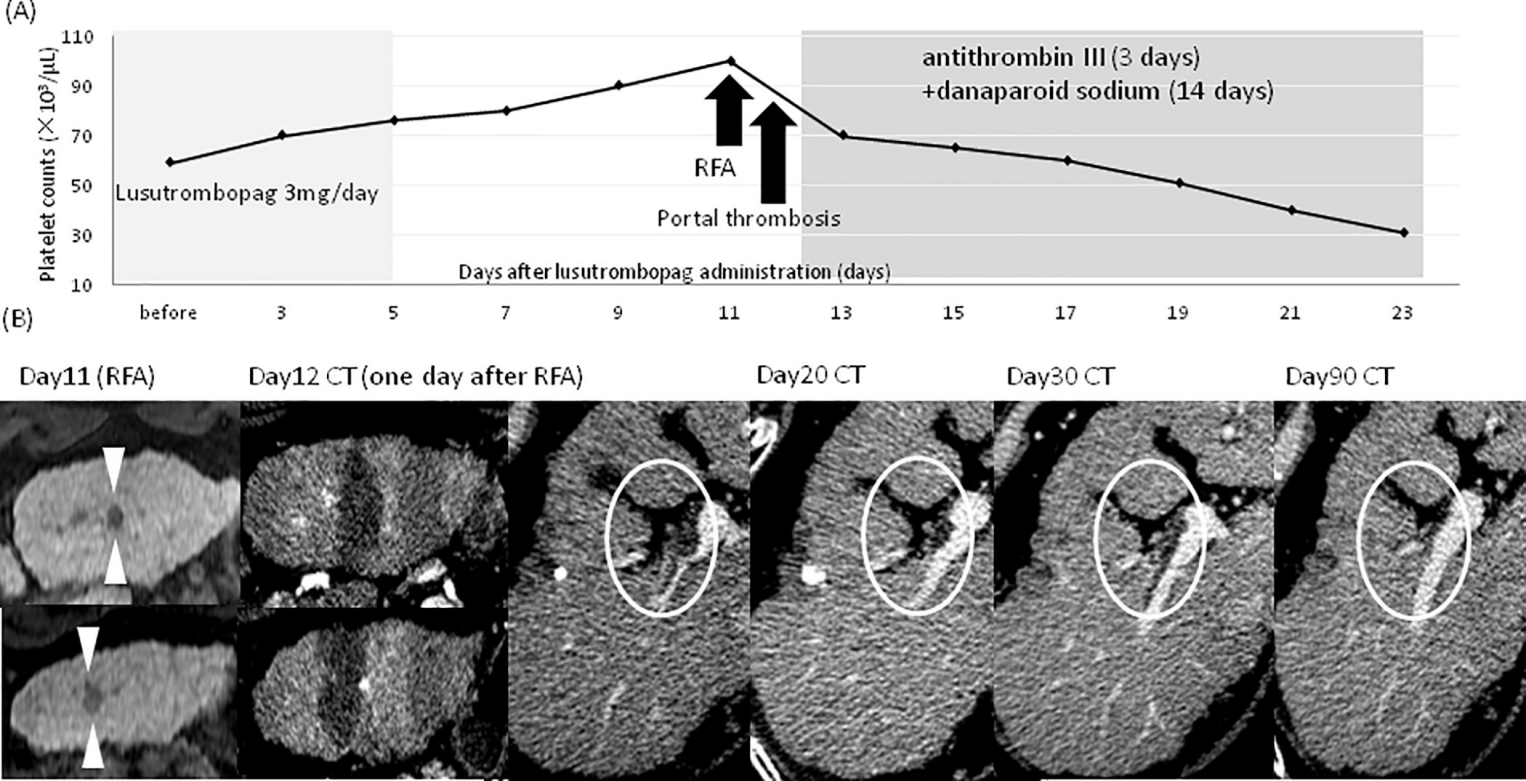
Detailed clinical course of a patient with portal thrombosis after lusutrombopag treatment. (A) Changes in platelet counts during and after lusutrombopag therapy. This patient had a prior history of portal thrombosis. Initially, 3 mg of lusutrombopag was administered from day 1 to day 5. Since the platelet count increased to 76,000/μL on day 5, the administration of lusutrombopag was discontinued. After RFA, portal thrombosis was detected and antithrombotic therapy with antithrombin III (for 3 days) plus danaparoid sodium (for 14 days) was given which resulted in the complete resolution of portal thrombosis. (B) Course of image findings. The arrowheads show HCC recurrence in the third segment of the liver. Dynamic CT imaging on day 12 shows the areas of ablation by RFA. Portal thrombosis occurred on CT on day 12 of lusutrombopag therapy requiring antithrombotic therapy. Complete resolution of thrombosis on day 90. HCC: hepatocellular carcinoma, RFA: percutaneous radiofrequency ablation.
